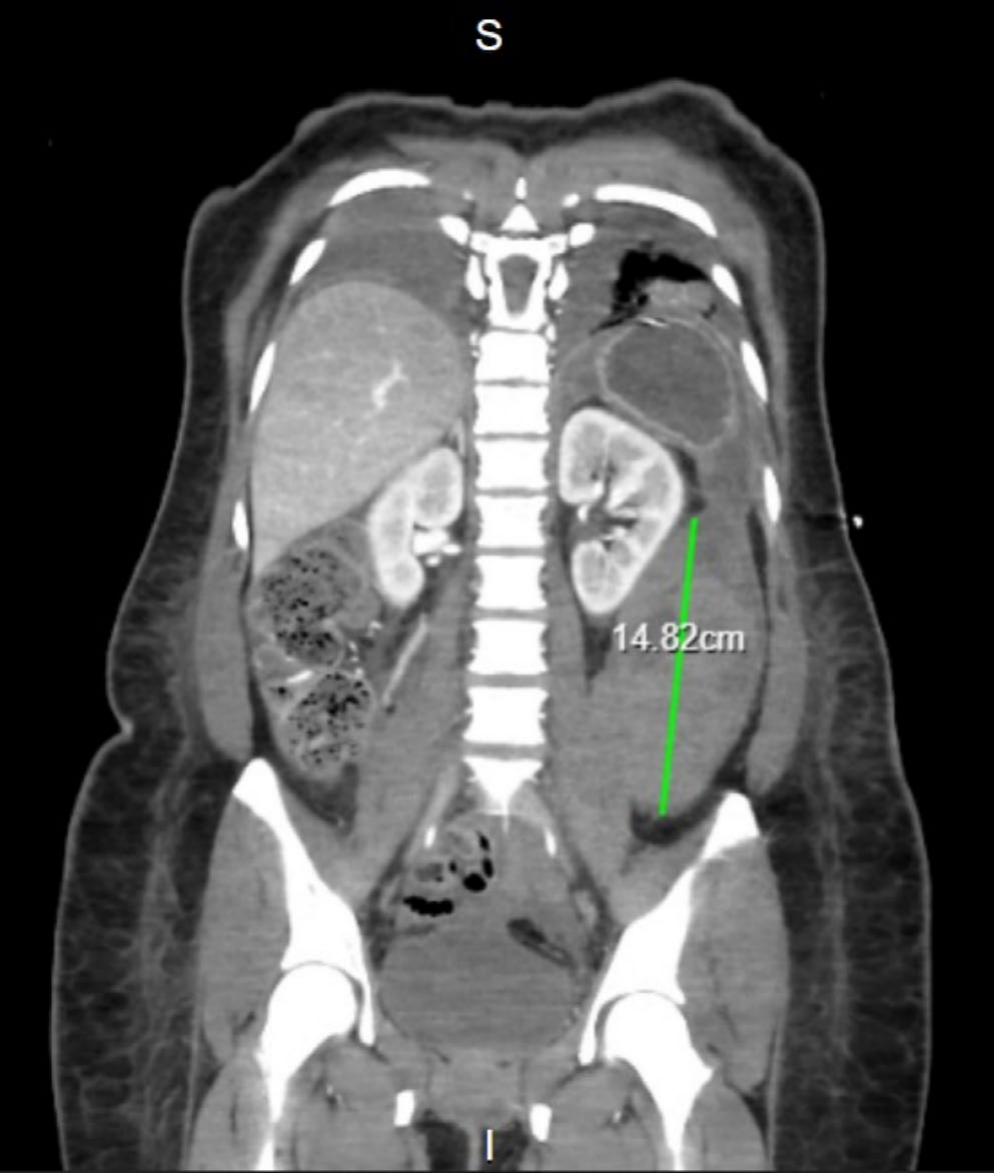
CT abdomen and pelvis performed on day 6 postoperatively, identifying a large left retroperitoneal haematoma, medial to the left iliopsoas muscle and inferior to the left kidney, in keeping with a haematoma, with no evidence of active bleeding.
